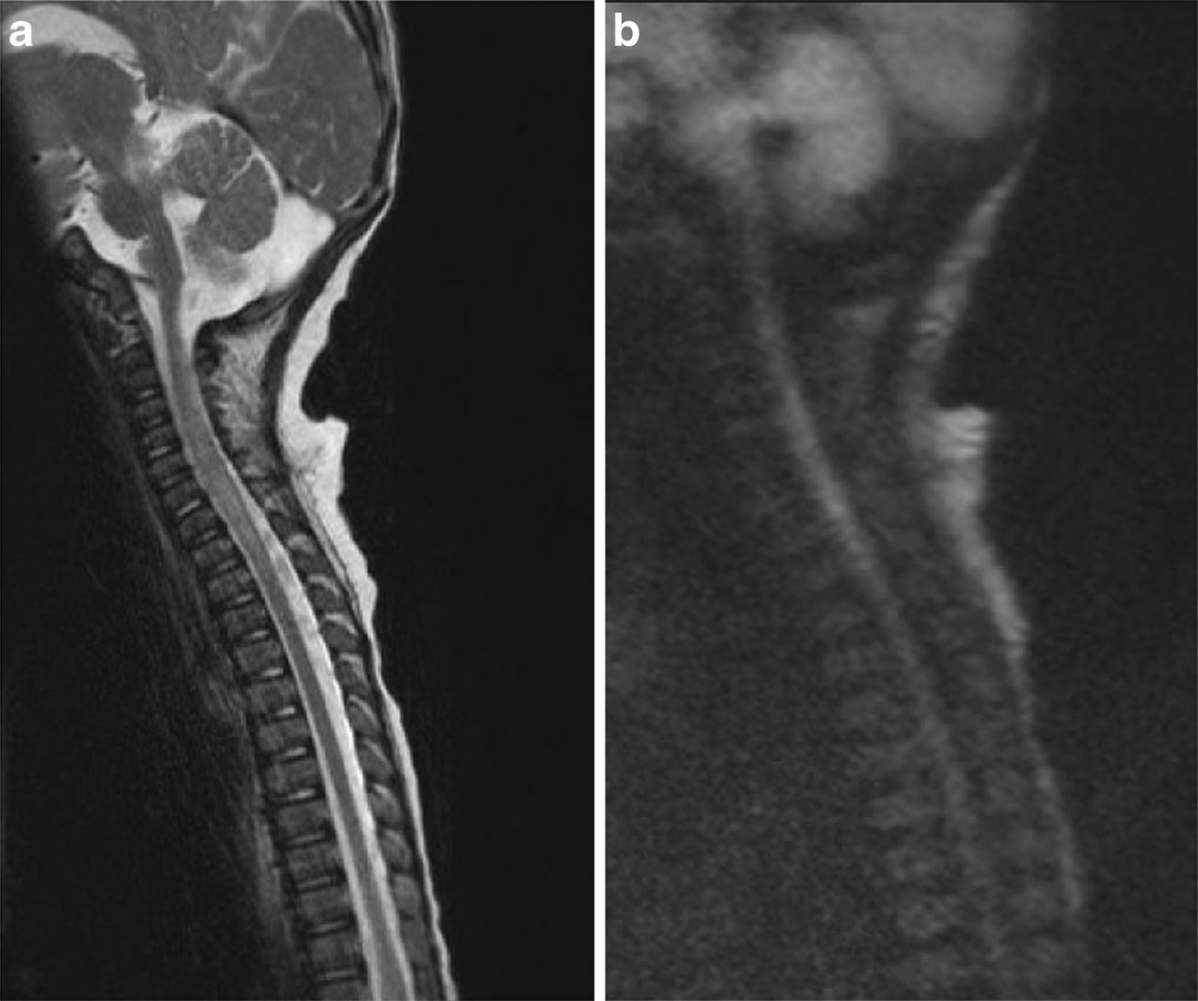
Repeat MRI assessment 2 days later confirmed the cord signal abnormality on standard T2 imaging (a) and showed concomitant apparent restriction on HASTE diffusion weighted imaging (DWI) (b) supporting a suspected acute ischaemic insult
